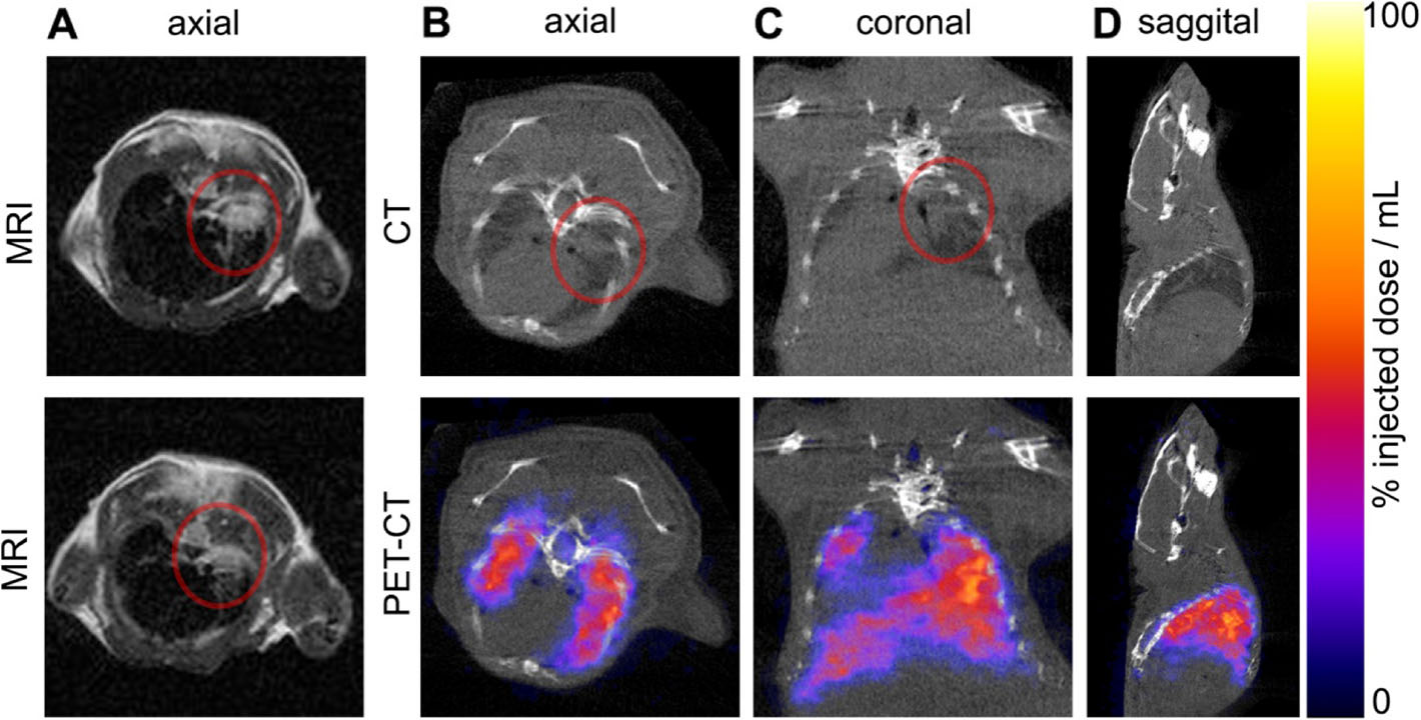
Tumour visualisation with MRI and CT and location of ^89^Zr-oxine-labelled MSCTRAIL visualised with PET at 2 days post-transplantation. Lung tumours detected with a axial T_2_-weighted magnetic resonance imaging (T_2_ RARE TE = 55 ms, respiratory gated) showing two consecutive 1-mm-thick slices through the lung tumour (shown in the red circle) at 15 days post-implantation b axial, c coronal, and d sagittal CT slices, with corresponding PET overlay showing the location of ^89^Zr-oxine-labelled MSCTRAIL in the lungs and liver
